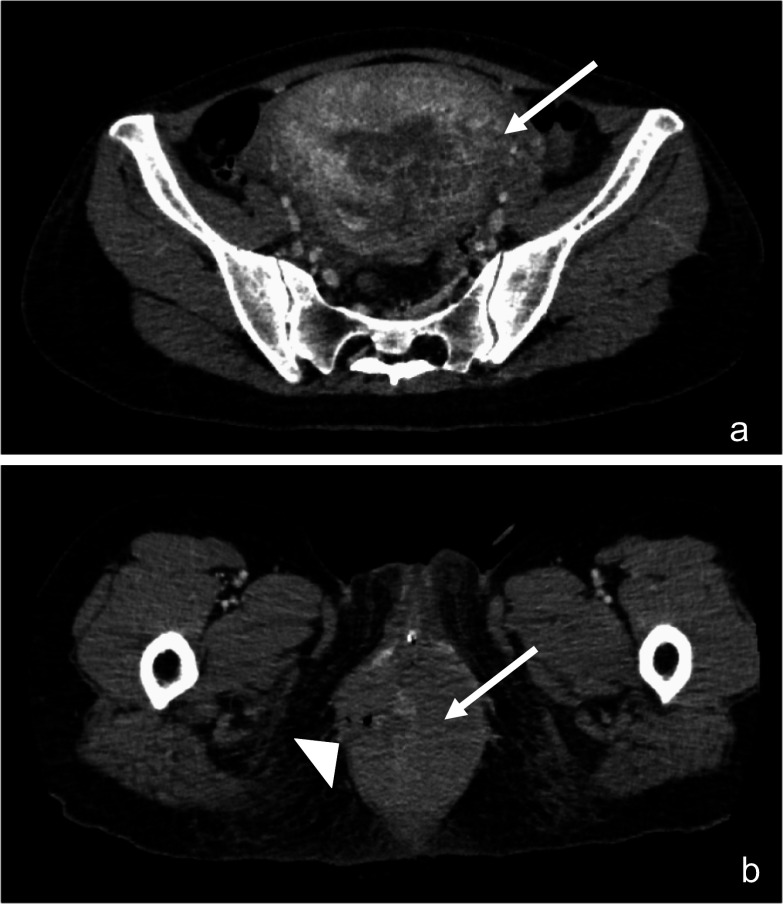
a A CT scan at the time of perineal laceration at delivery revealed a large amount of hematoma from the uterine cavity to the vagina (arrow). b A hematoma was also observed around the anus and rectum (arrowhead)
